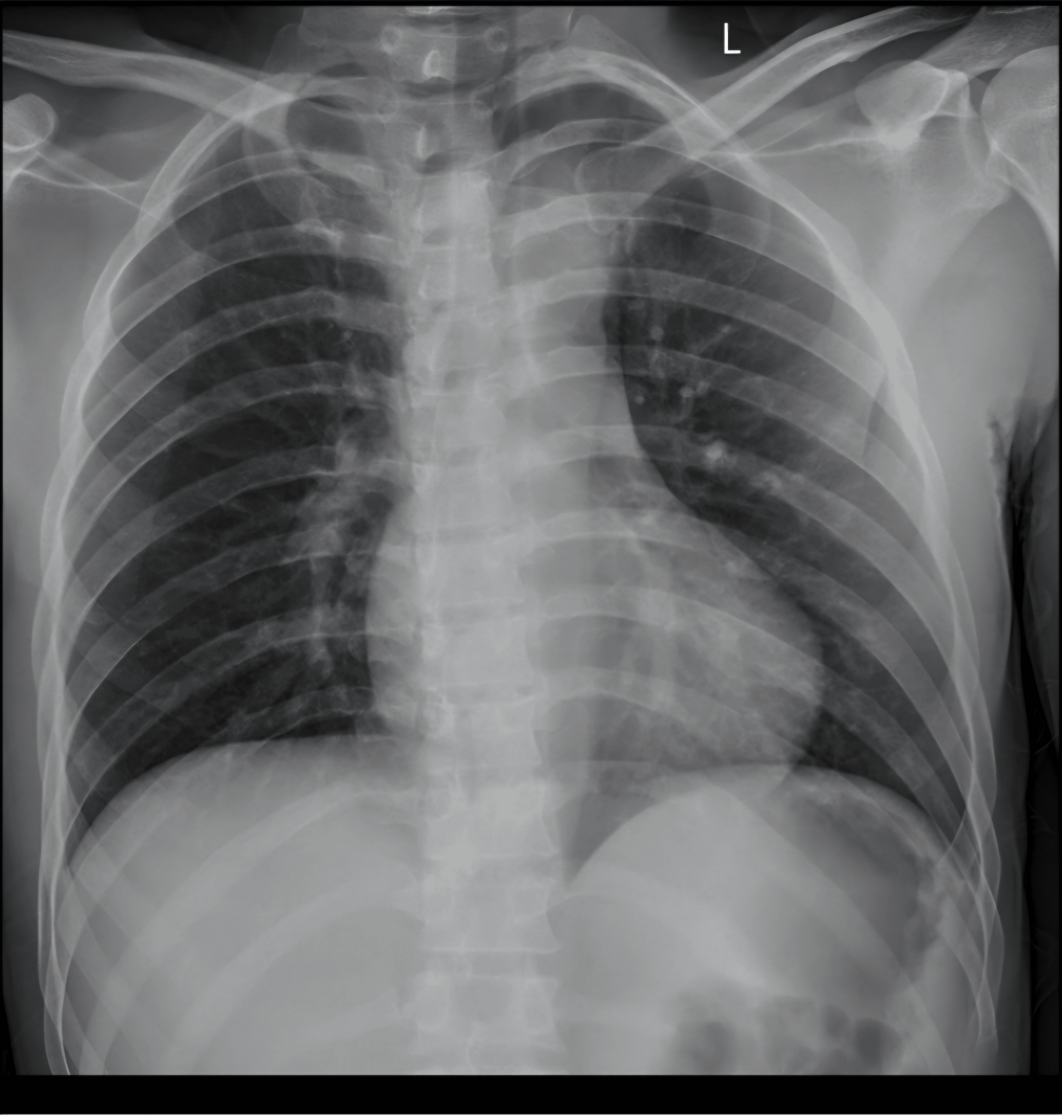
Chest radiograph with normal findings on the day of admission
